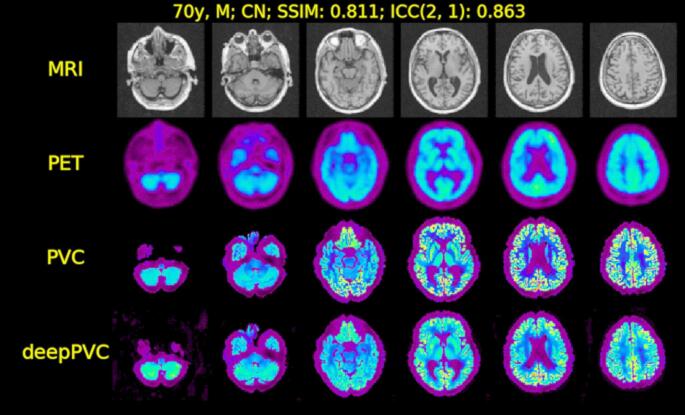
MR, PET, and both real and predicted PVC maps for instances with the highest ICC in the [^18^F]-FDG test data. CN stands for cognitively normal; MR for magnetic resonance; PET for positron emission tomography; and PV for partial volume. The deepPVC predictions show high similarity to reference PVC maps, with improved structural definition and contrast in cortical and subcortical regions. Reprinted with permission from [205] under a Creative Commons Attribution License (CC BY 4.0 DEED)
